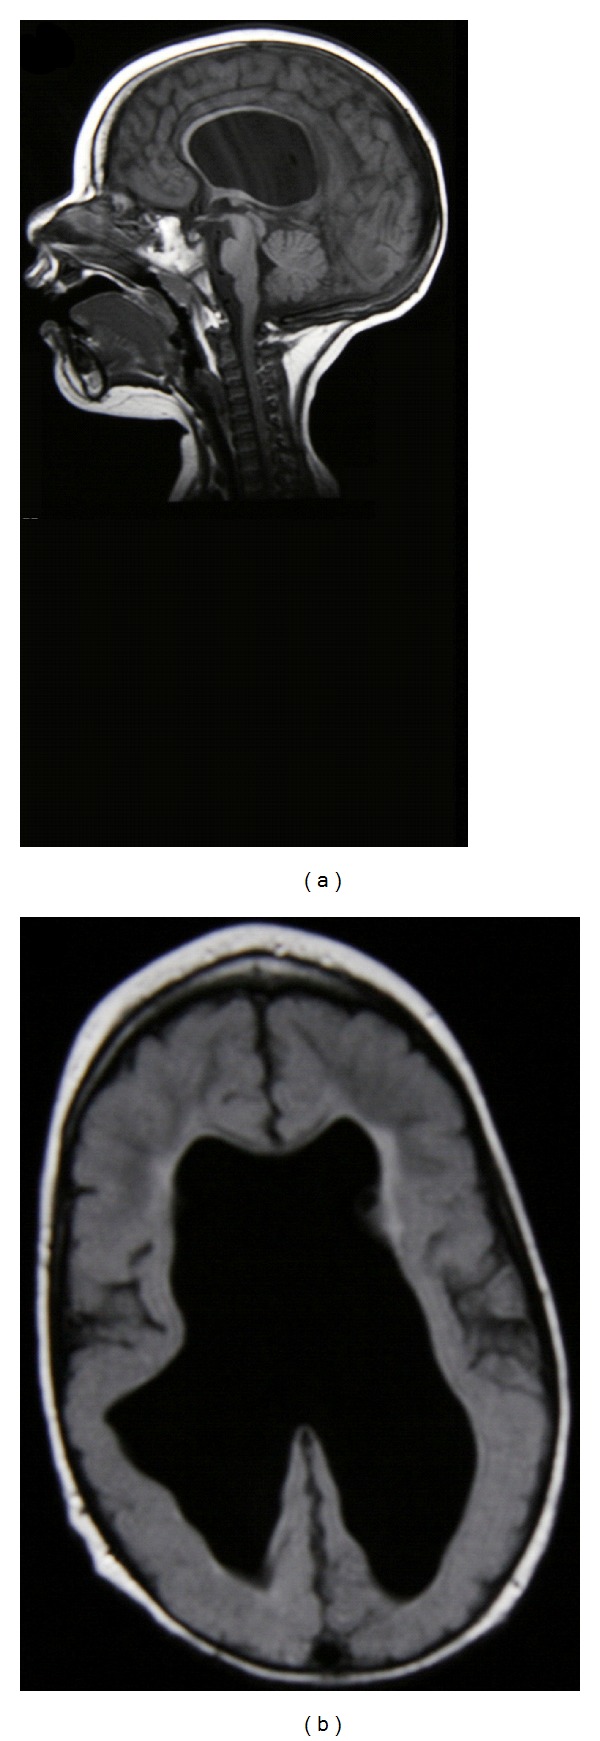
Sagittal T1 (a) and transverse FLAIR (b) MRI of sixth pregnancy (third case) in family 1. Note thin corpus callosum in (a). Irregular ventricular borders, white matter hypoplasia, ventriculomegaly, and periventricular hyperintense signal are demonstrated in (b).
